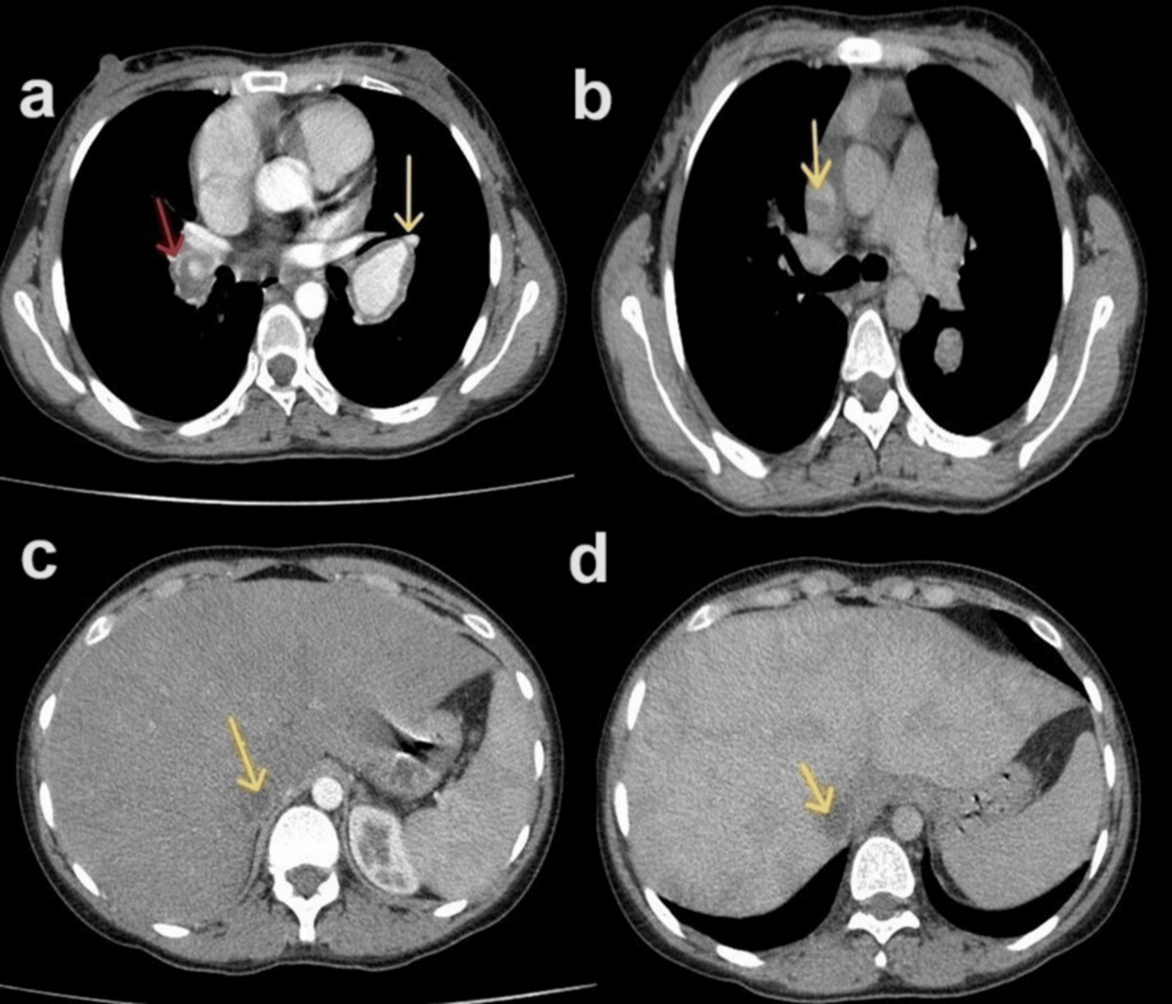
Computed tomography pulmonary angiography (CTPA): (a) Arterial phase shows right pulmonary artery aneurysmal dilatation with a filling defect representing thrombosis (red arrow) and left pulmonary artery dilatation with wall thickening (yellow arrow). (b) The late venous phase shows a filling defect in the superior vena cava (SVC), indicating SVC thrombosis (yellow arrow). (c) Arterial phase and (d) late venous phase show inferior vena cava (IVC) filling defects (yellow arrows) with hepatomegaly and heterogeneous enhancement (nutmeg liver), indicating an association with Budd-Chiari syndrome.
